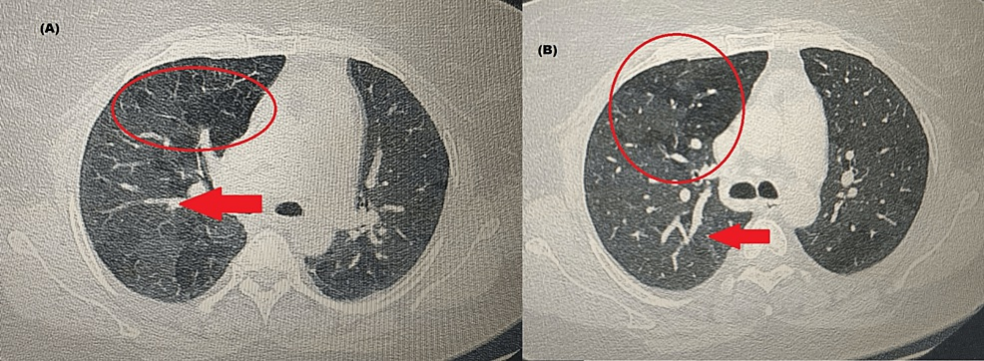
High-resolution CT (HRCT) chest after three months follow-up at two different CT cuts Both (A) and (B) show resolving mosaic attenuation (encircled) in bilateral lung fields and tractional bronchiectasis (arrow) with adjacent fibrocystic and fibroconsolidatory changes
